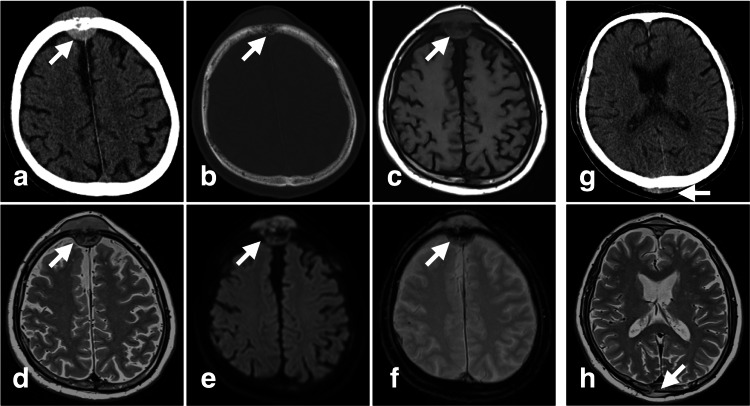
CT and MRI before CKR The frontal bone lesion (white arrows): (a) plain CT, (b) bone CT, (c) T1WI, (d) T2WI, (e) DWI, (f) T2*. The occipital bone lesion (white arrows): (g) plain CT, (h) T2WI CT: computed tomography, MRI: magnetic resonance imaging, CKR: CyberKnife radiotherapy, bone-CT: bone window computed tomography, T1WI: T1-weighted image, T2WI: T2-weighted image, DWI: diffusion-weighted image
